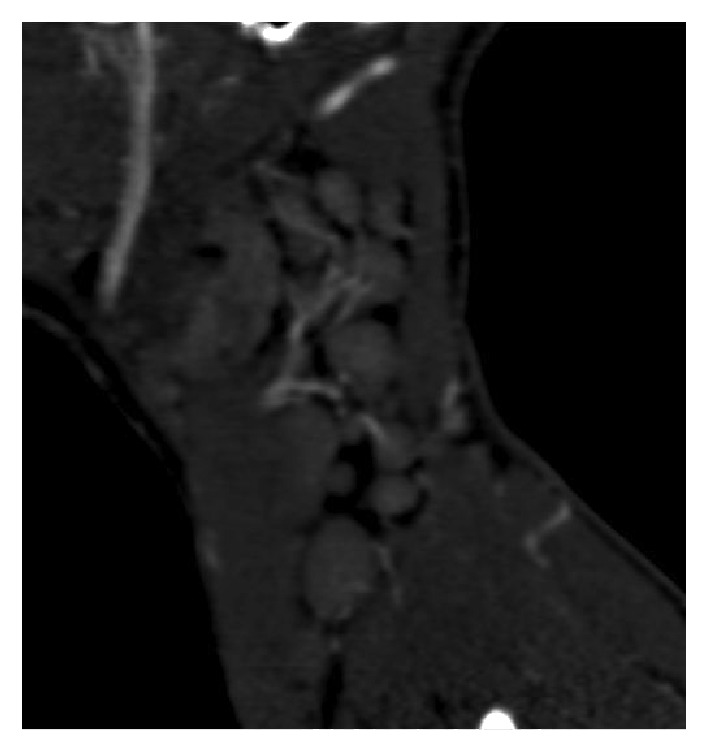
Sagittal CT angiogram demonstrating extensive hypervascularity of lymph nodes in the left neck.
